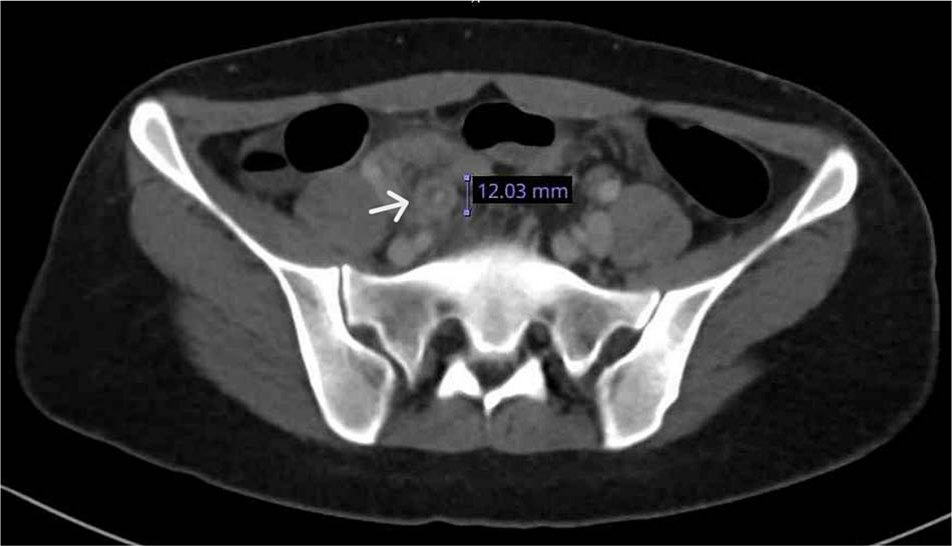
CT axial plane demonstrated a thickened appendix (white arrow) in the right iliac fossa measuring 12 mm.
